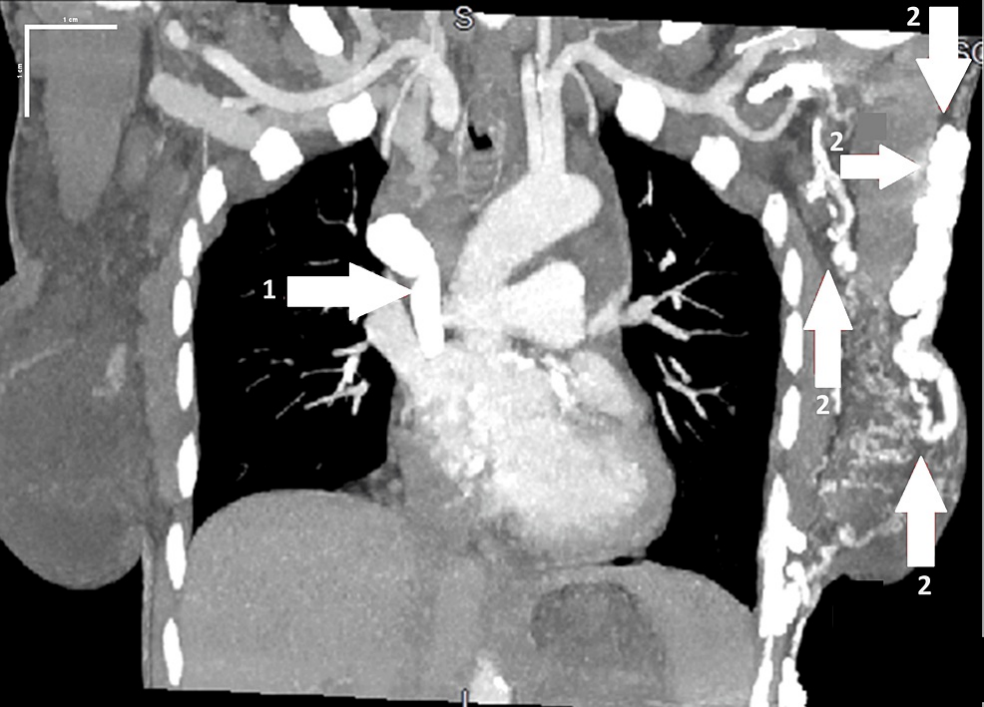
Coronal section computed tomography angiography (scale bar, 1 cm) showing via label 1: a hypertrophied azygos vein and via label 2: hypertrophied venous collateral vessels along the left chest wall.
